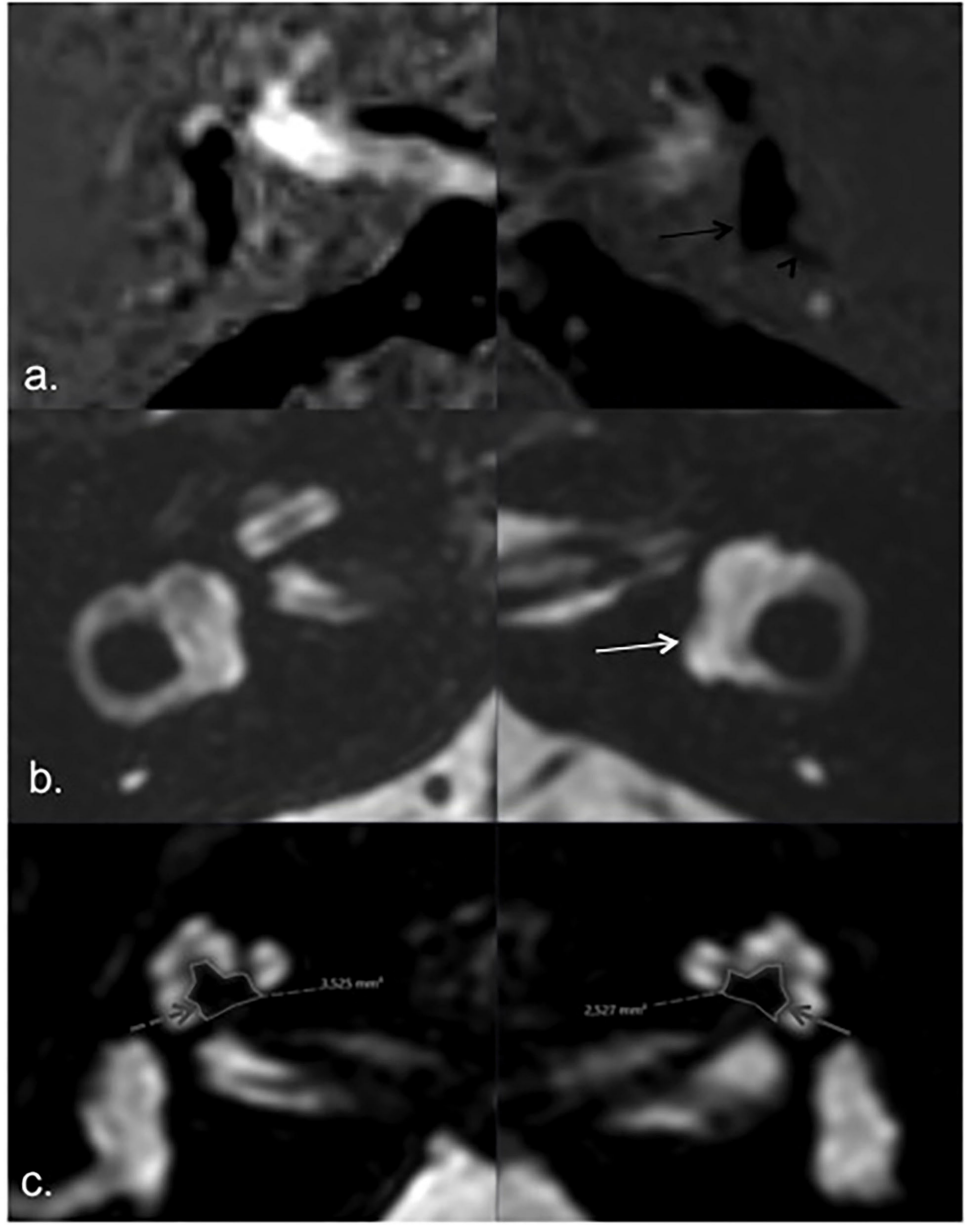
MRI of patient 1. HYDROPS (a) and T2 (b) axial images showing endolymphatic (black arrow) and total membranous labyrinth (white arrow) respectively; bilateral vestibular endolymphatic hydrops, more pronounced on the left side (a. black arrow) with herniation of the utricle into the non-ampullary limb of the lateral semicircular canal (black arrowhead). Axial T2 image according to the greater axis of cochlear modiolus (c), showing marked asymmetric modiolar areas, smaller on the left side (index ear) (solid gray arrow) compared to the left asymptomatic side (dashed gray arrow).
